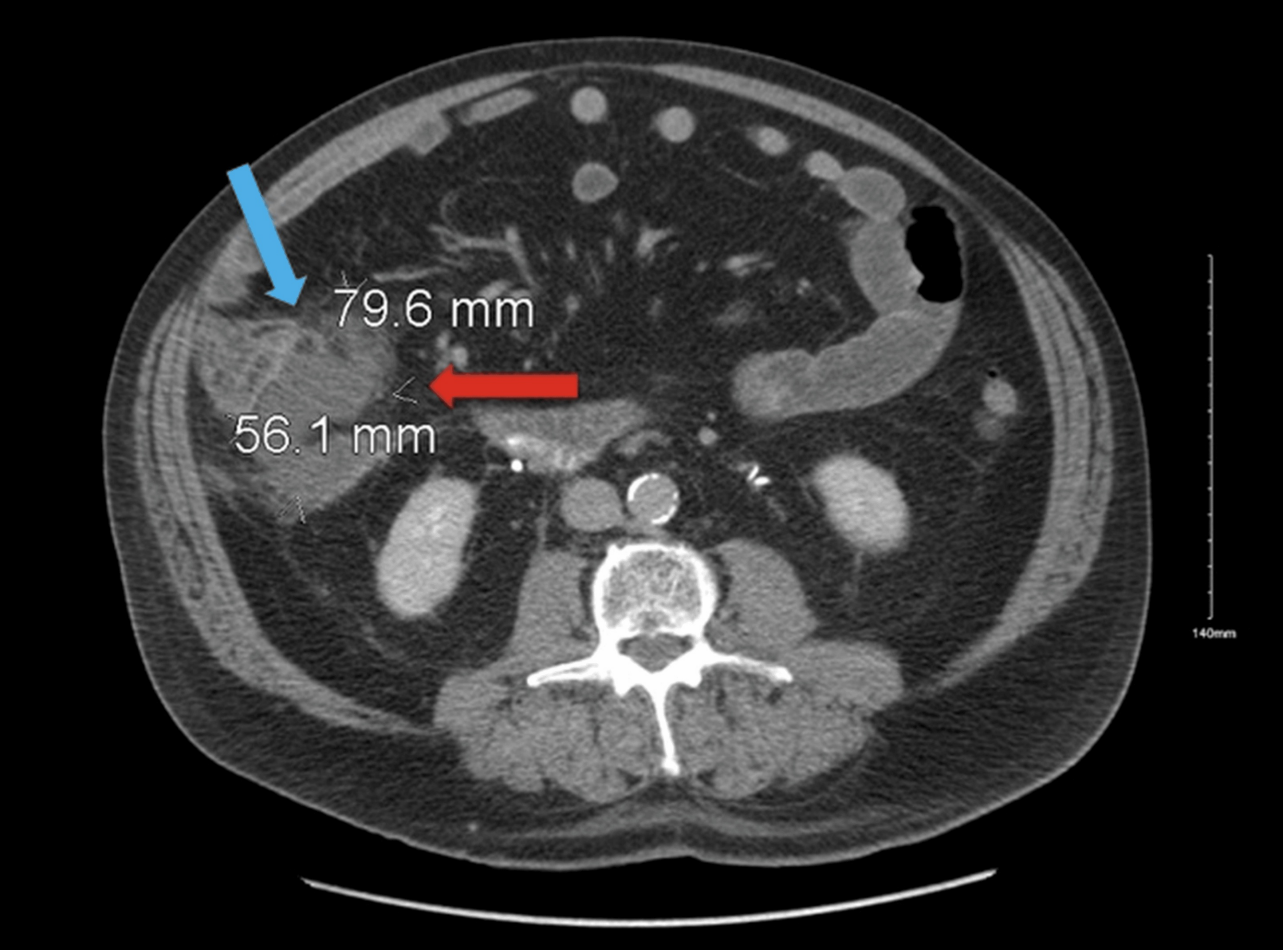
Contrast-enhanced computed tomography (CT) of the abdomen and pelvis (supine view) showing pneumobilia (blue arrow) and a pericholecystic fluid collection (red arrow) consistent with a contained gallbladder perforation. The collection measured approximately 8 × 5.6 cm and extended from the base of the appendix toward the right upper quadrant.
